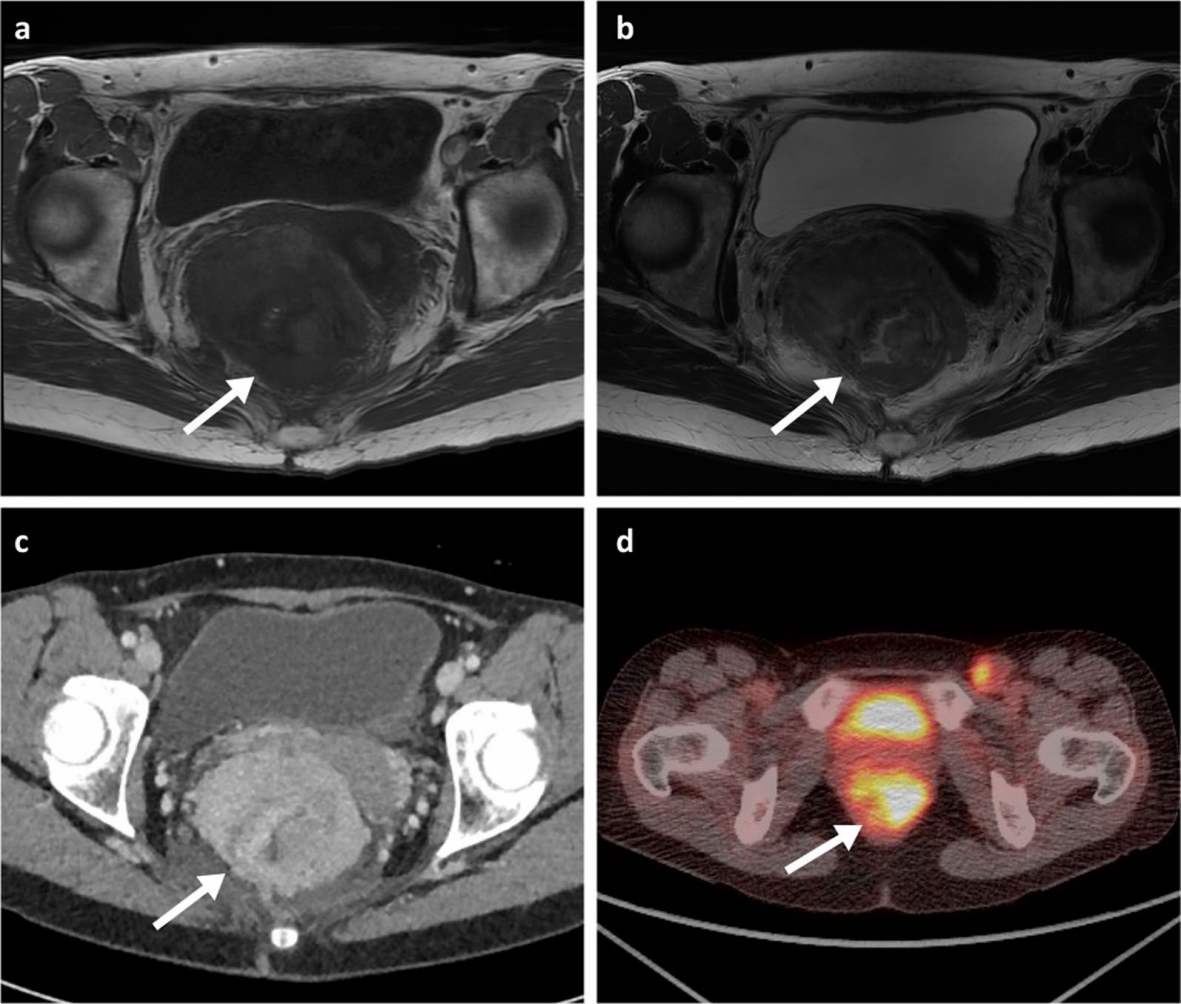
MRI T1WI and T2WI axial (a, b), portal phase contrast-enhanced CT axial (c) and PET-CT axial (obtained two months prior to the shown MRI and CT) (d) of a 57-year-old female patient with a large metastatic melanoma anorectal mass (arrows). The mass demonstrates internal foci of higher T1 signal. PET uptake is also seen within a left inguinal node
